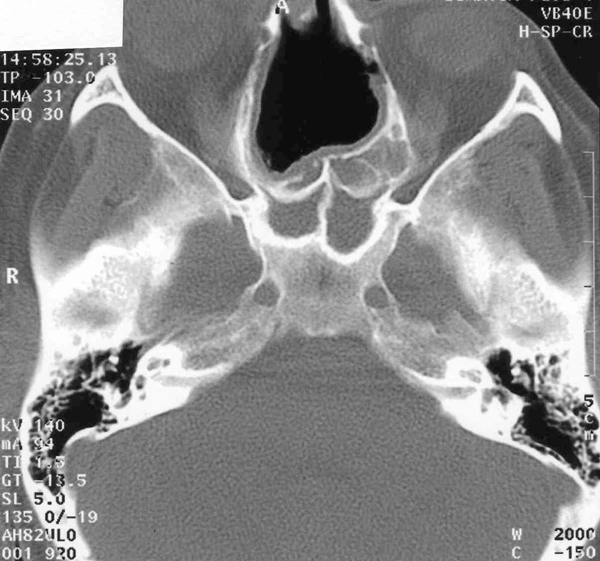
CT scan of the sinuses demonstrating a large septal perforation.
